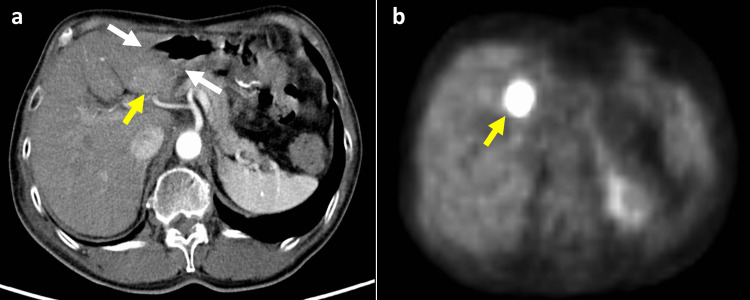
Contrast-enhanced CT and PET-CT after four courses of chemotherapy. Contrast-enhanced CT (a) and PET-CT (b) after four courses of chemotherapy showed new liver metastasis in the S2 liver (yellow arrow). This recurrence lesion was located adjacent to the gastrointestinal tract (white arrow).
